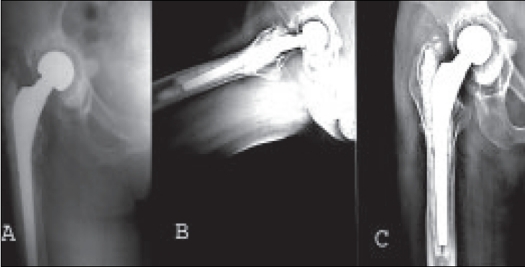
Ten years follow-up X-rays of a 65-year-old female showing osteolysis in all seven femoral and all three acetabular zones but clinically patient was asymptomatic
